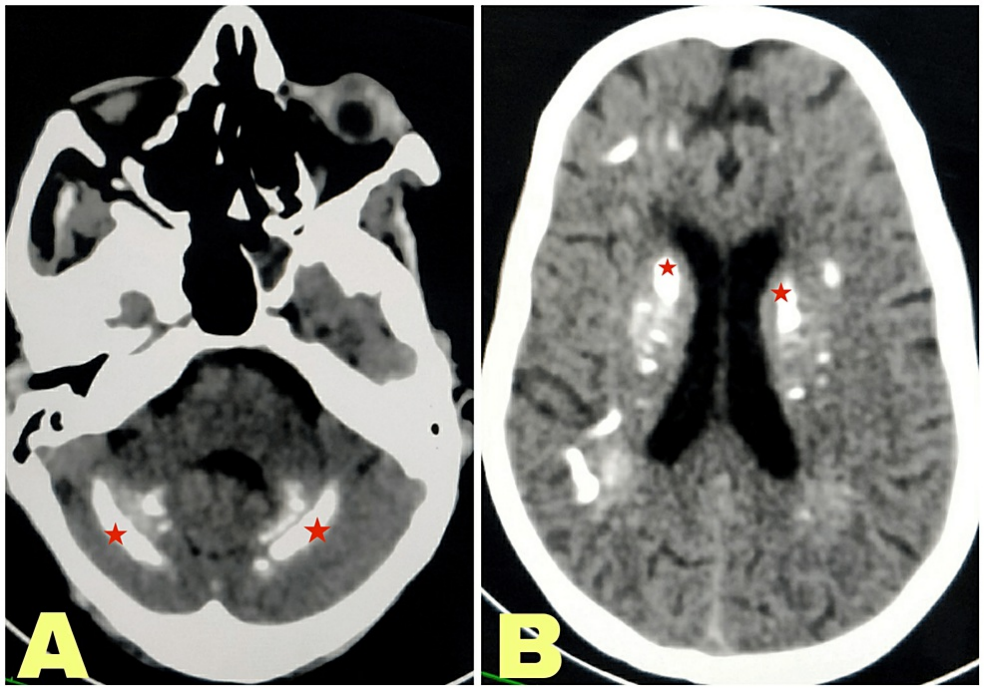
(A) Axial non-contrast CT scan at the level of cerebellum showing bilateral calcification in the region of cerebellar cortex (red star); (B) Axial non-contrast CT scan at the level of basal ganglia demonstrating bilateral calcification in the region of caudate nucleus, globes pallidum, and putamen (red star)
